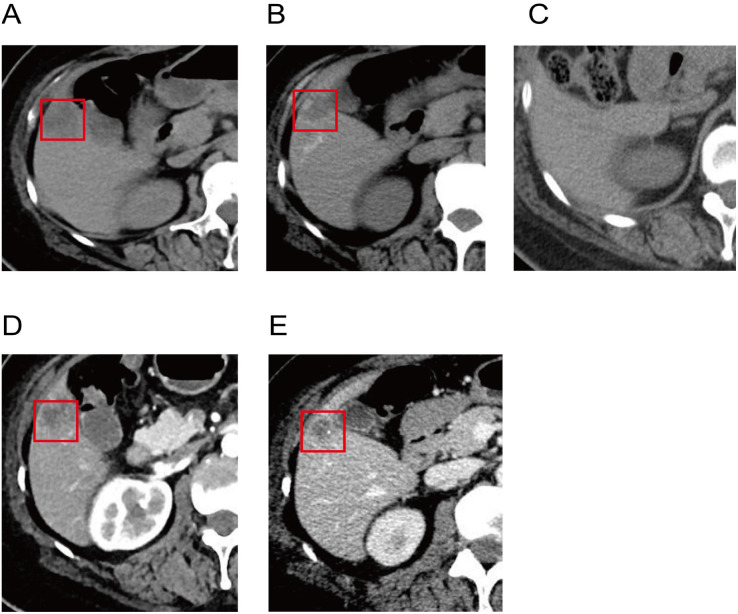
Serial abdominal CT demonstrating treatment response and tumor devascularization. Upper row: Non-contrast CT. (A) Pre-TAE (May 2, 2021): Hypodense lesion in hepatic segment V (3.0 × 2.7 cm, red box) with central necrosis. (B) Approximately 7 weeks after first TAE (June 28, 2021): Lesion decreased to 2.2 × 1.5 cm (red box) with lipiodol deposition. (C) Approximately 5 months after second TAE (November 10, 2021): Further regression to 1.7 × 1.2 cm. Lower row: Contrast-enhanced CT. (D) Pre-TAE (May 2, 2021): Hepatic segment V lesion (red box) with peripheral rim enhancement, indicating arterial blood supply. (E) Approximately 7 weeks after first TAE (June 28, 2021): Marked reduction in arterial enhancement (red box), consistent with successful tumor devascularization following embolization.
